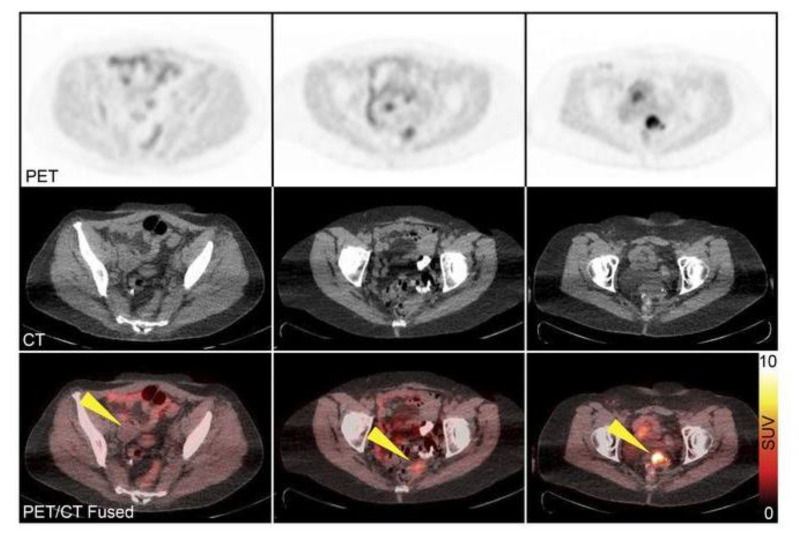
PET/CT images of ^18^F-FTT uptake in ovarian cancer patients. PET/CT images from a clinical trial (NCT02637934) in three ovarian cancer patients show a wide range of ^18^F-FTT uptake in tumor lesions. Standard uptake value (SUV) ranges from 2 (top-left) to 12 (top-right). Yellow arrows indicate sites of tumor. Reproduced with permission from Makvandi et al., titled “A PET imaging agent to evaluate PARP expression in ovarian cancer”, published by The Journal of Clinical Investigation, 2018 [46].
